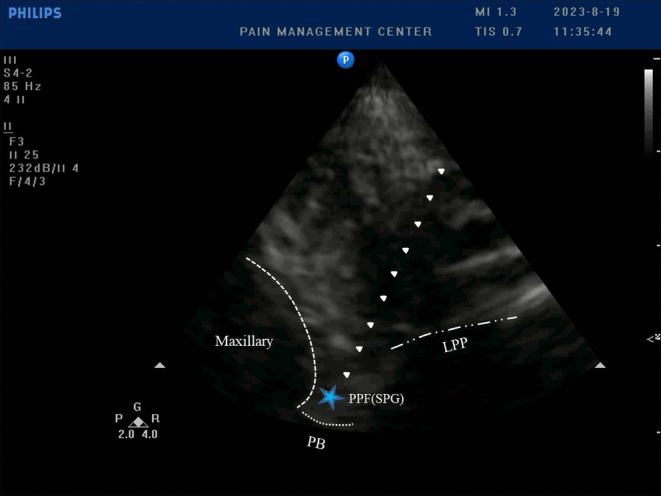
Ultrasound‐guided percutaneous puncture of the SPG by subzygomatic arch approach. After assisting the child to open her mouth, an ultrasound image of the pterygopalatine fossa was visible. The white arrows indicated the trajectory of the radiofrequency cannula and the blue pentagram represented the location of the SPG. PB, palate bone; PPF, pterygopalatine fossa; LPP, lateral pterygoid plate.
